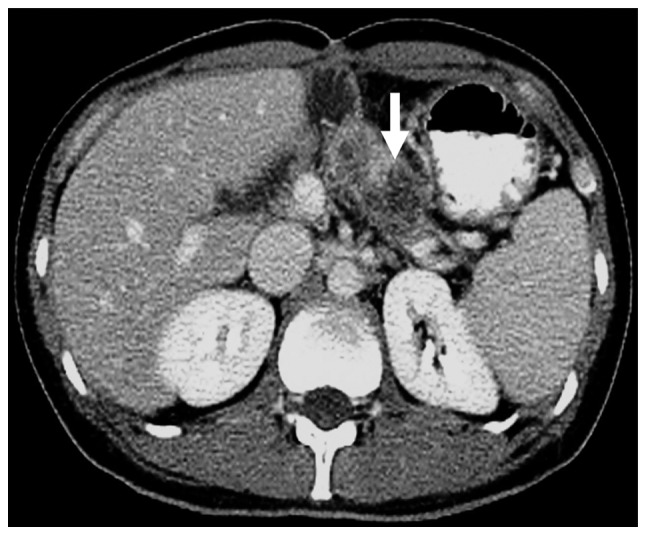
Enhanced abdominal computed tomography conducted four years following the initial surgery revealed a mass (diameter, 2 cm) in the remnant pancreas (arrow).
